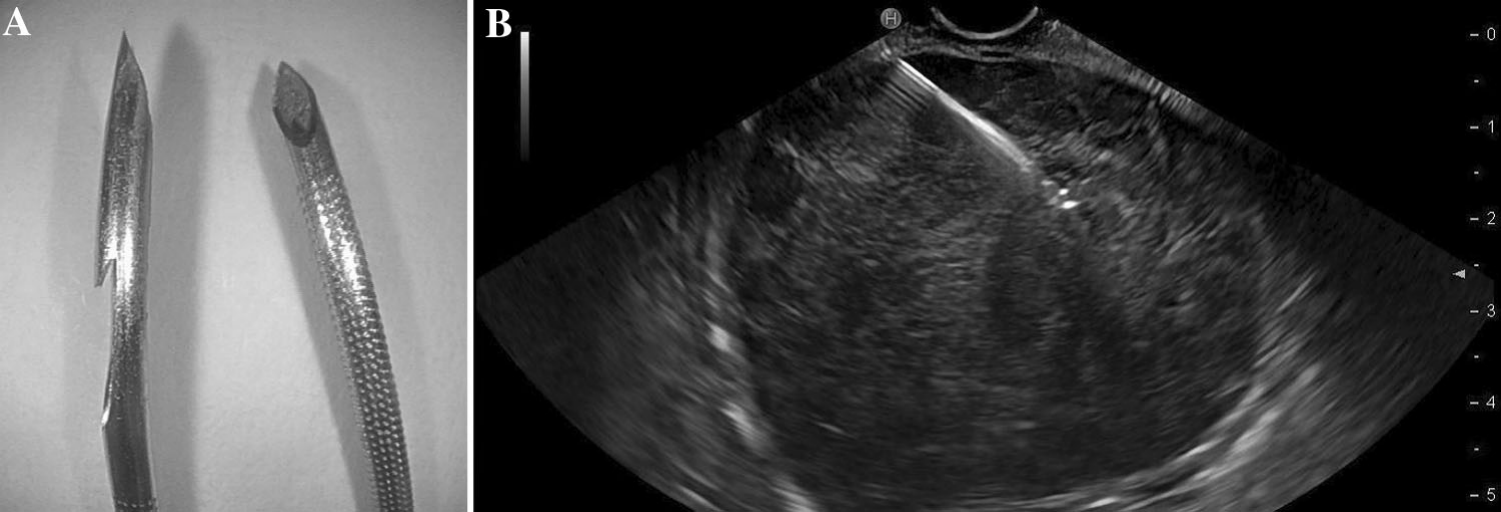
Left image (A) a close-up photo showing the tip of the 22 gauge FNB-needle with the side fenestrated, reverse bevel design (*left*) and the tip of a standard 25 gauge FNA-needle (*right*). The photo was captured with a Fujifilm HD Video Endoscope EG760Z (Tokyo, Japan). Right image (B) the endosonography image of a 6 cm hypoechoic gastrointestinal stromal tumor situated in the gastric body during EUS-guided sampling with a 22 gauge reverse bevel FNB-needle
